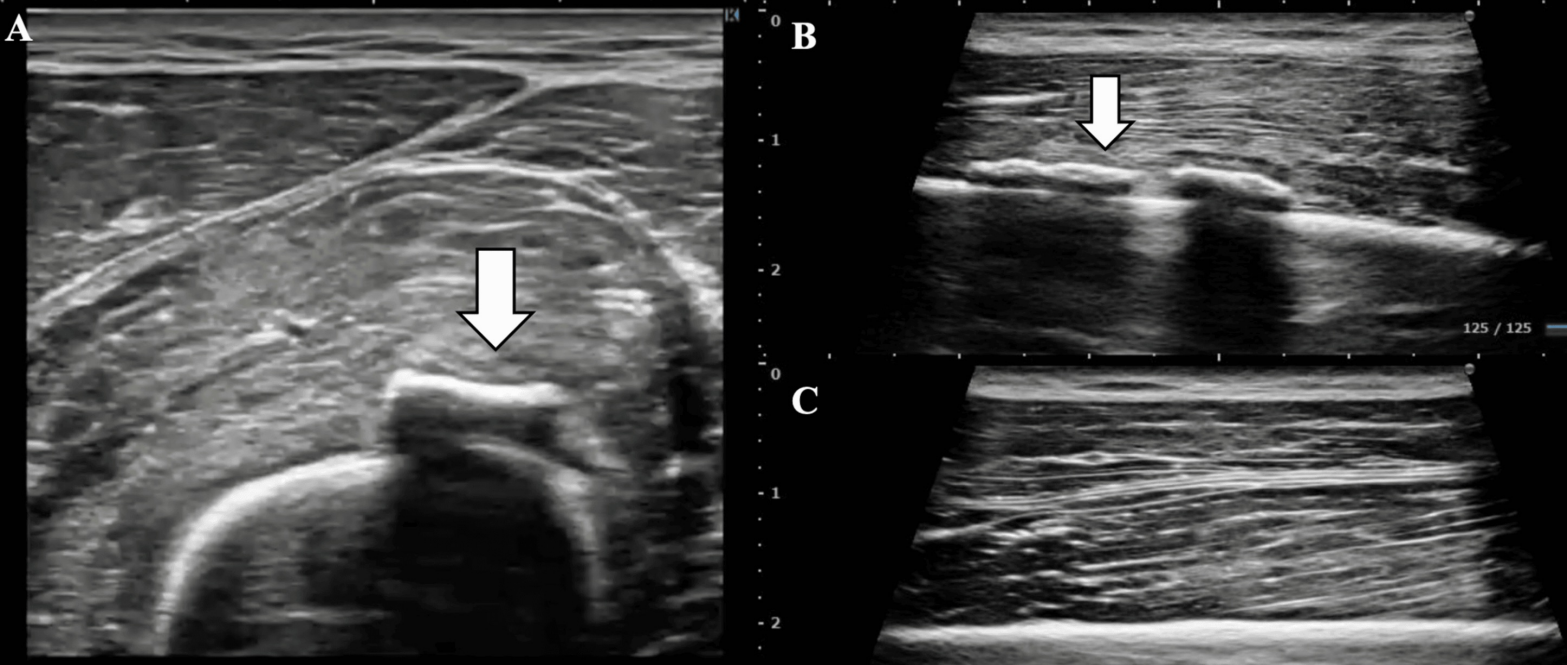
Follow-up ultrasound image of the thigh (A) Short-axis and (B) long-axis ultrasound image of the vastus intermedius on the affected side. (C) Long-axis ultrasound image of the vastus intermedius on the unaffected side Arrow: Heterotopic ossification observed within the vastus intermedius
